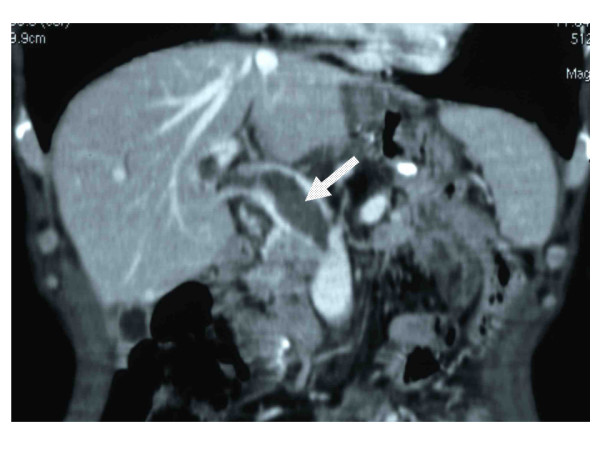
Contrast-enhanced abdominal CT. The thrombosis located in the superior mesenteric vein with portal vein (arrow) without cavernous formation.
